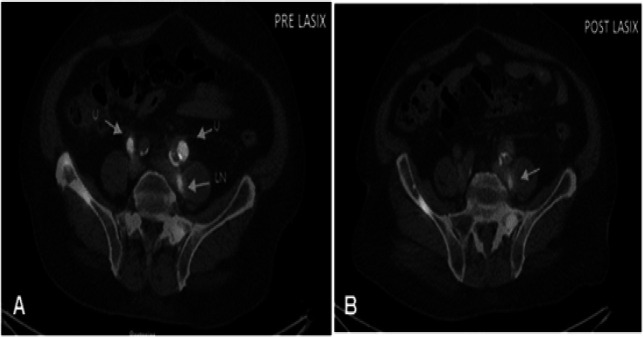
Transaxial Fused PET/CT Images (A) of the pelvis showing three focal lesions the in pre Lasix study (arrows), However, post Lasix images (B) showed clearance of activity from two areas labeled as ‘U’ leaving behind the true uptake in the lymph node (LN)
